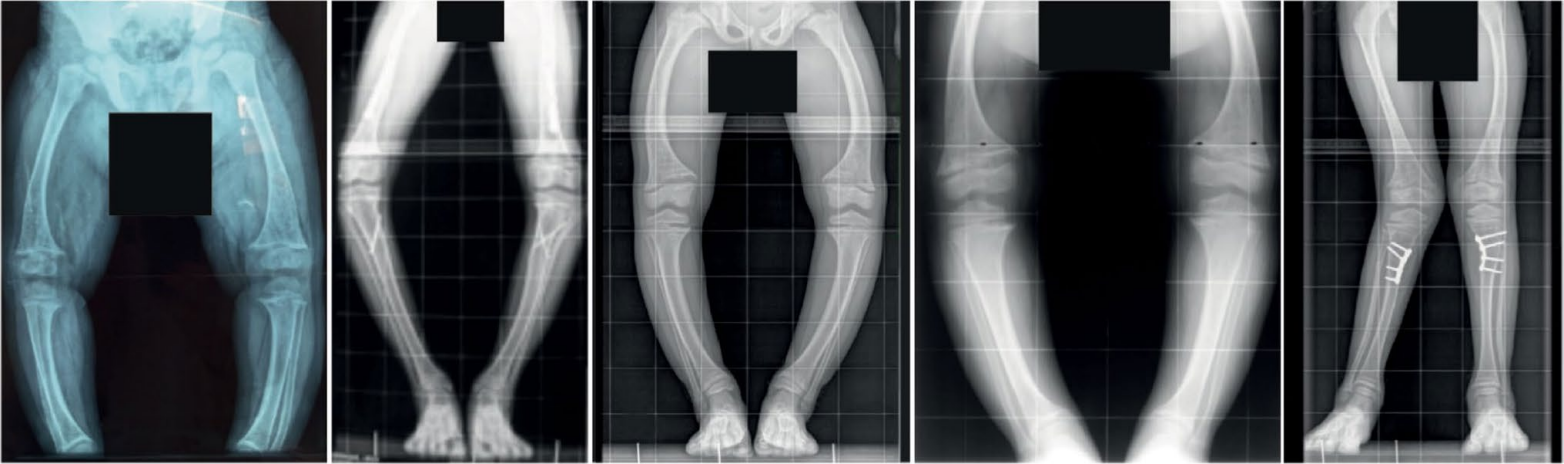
Radiographs of the lower extremities of children with X-linked hypophosphatemia. The patients show disproportionate short stature with genu varum (bowed legs). The radiographs reveal severe leg bowing, partial fraying, and irregularity of the distal femoral and proximal tibial growth plates. Note the lack of bone resorption features. Reproduced with permission from ref. [9]
